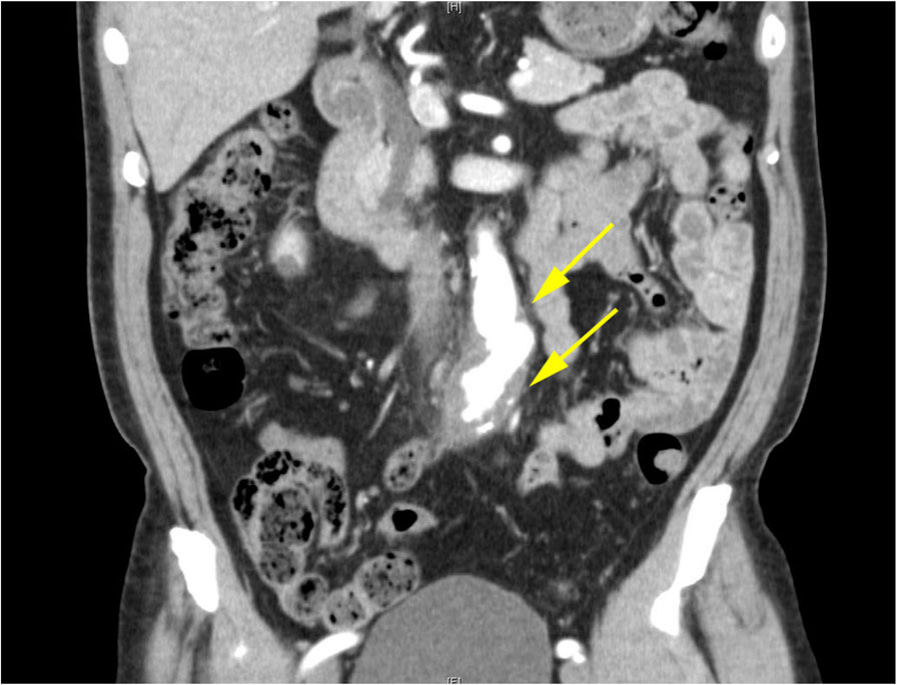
Coronal contrast-enhanced CT abdomen image demonstrates irregular wall thickening of the descending aorta having a wild, multilobulated appearance with surrounding soft tissue stranding
